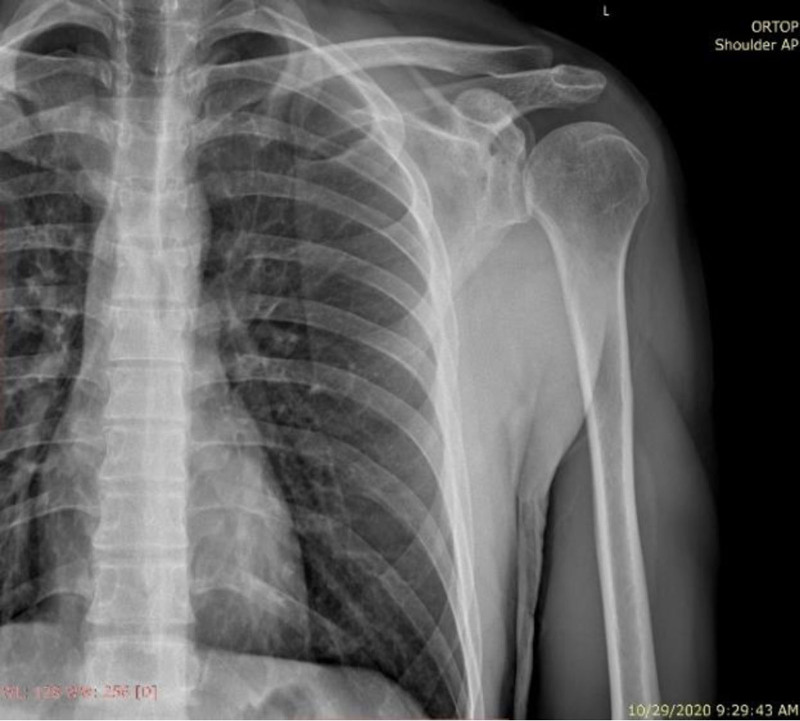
Anteroposterior X-ray at 2-year follow-up.
